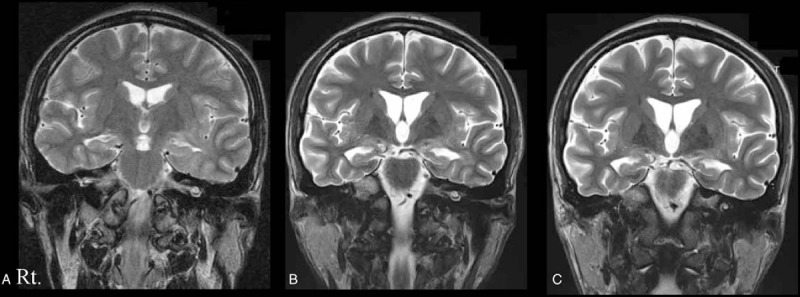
Serial MRI studies in Patient 3. The first brain MRI on day 1 was normal (panel A). Diffuse brain atrophy was evident on the second brain MRI, obtained on day 14 (panel B). Brain atrophy was evident on the final follow-up MRI, obtained 64 days after admission (panel C).
